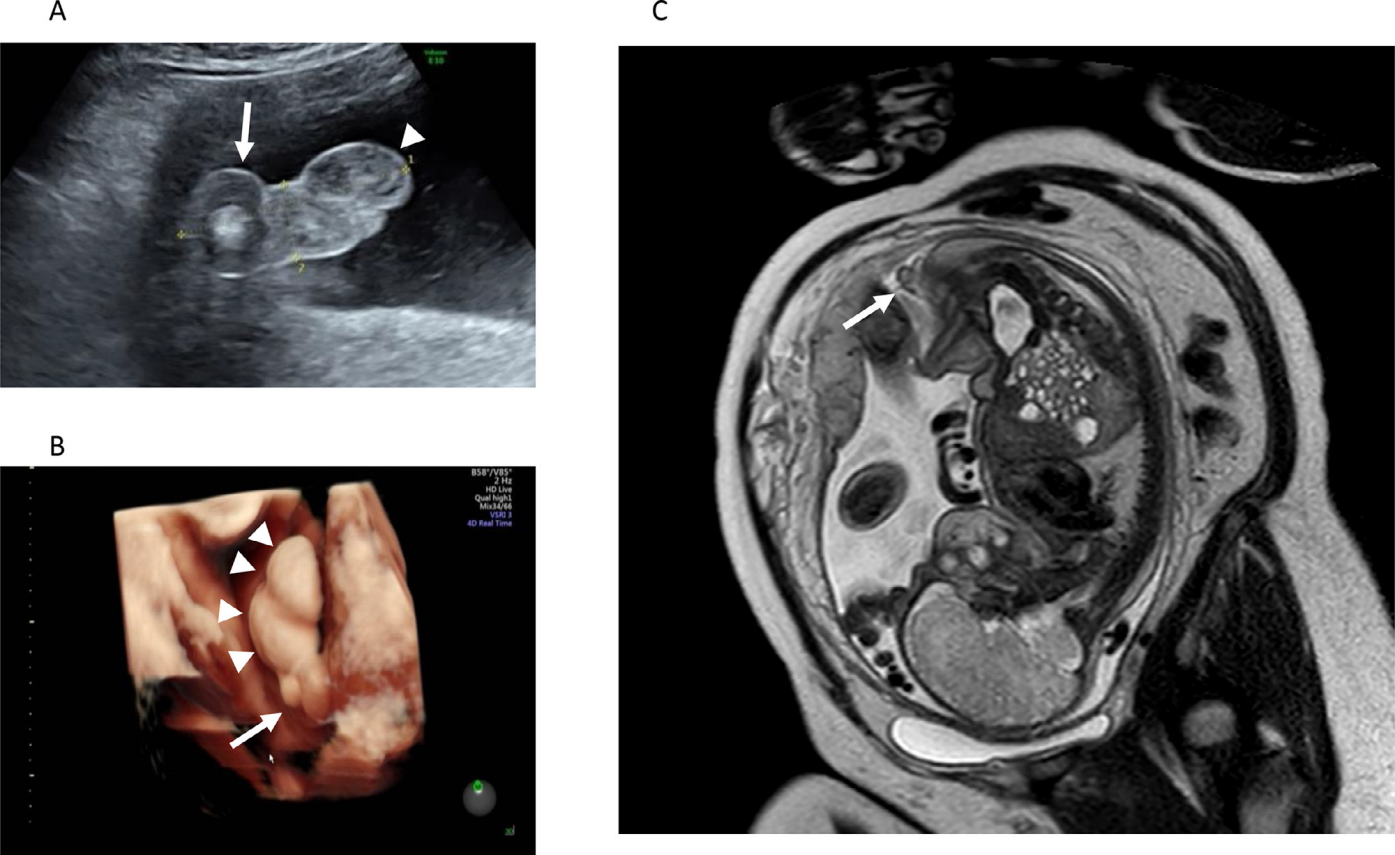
Prenatal images. (A) A prenatal ultrasonography on an axial perineal view of the perineum at 35 weeks of gestation shows a 2.0-cm-sized circular mass (arrow) posterior to the normal scrotum (arrow head). (B) The three-dimensional reconstruction image of ultrasonography shows a mass (arrow) located posterior to the normal scrotum (arrowheads). (C) A prenatal magnetic resonance image shows a perineal mass with adipose tissue characteristics (arrow).
